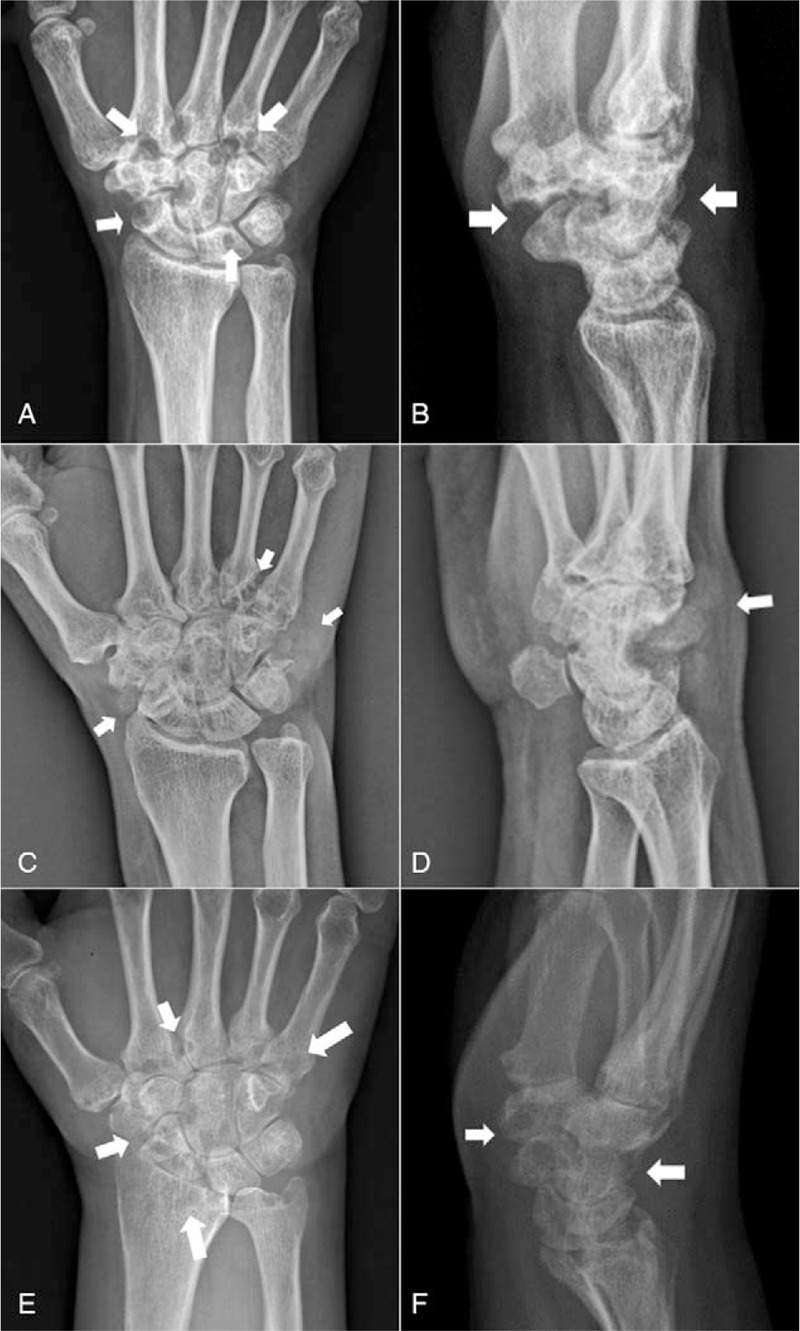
Plain x-ray, anteroposterior (AP), and lateral views of the 3 patients. Plain radiographs of Case 1 (A and B) reveal multiple well-defined carpal and metacarpal base erosions (arrows). In Case 2, the AP (C) and lateral views (D) show multiple erosions of the carpal bones and metacarpal bases, with surrounding, ill-defined, lobulated soft tissue density (arrows). Case 3 (E and F) also reveals multiple erosions of the radius, carpal bones, and metacarpal bases (arrows), with narrowing of the radiocarpal joint space.
